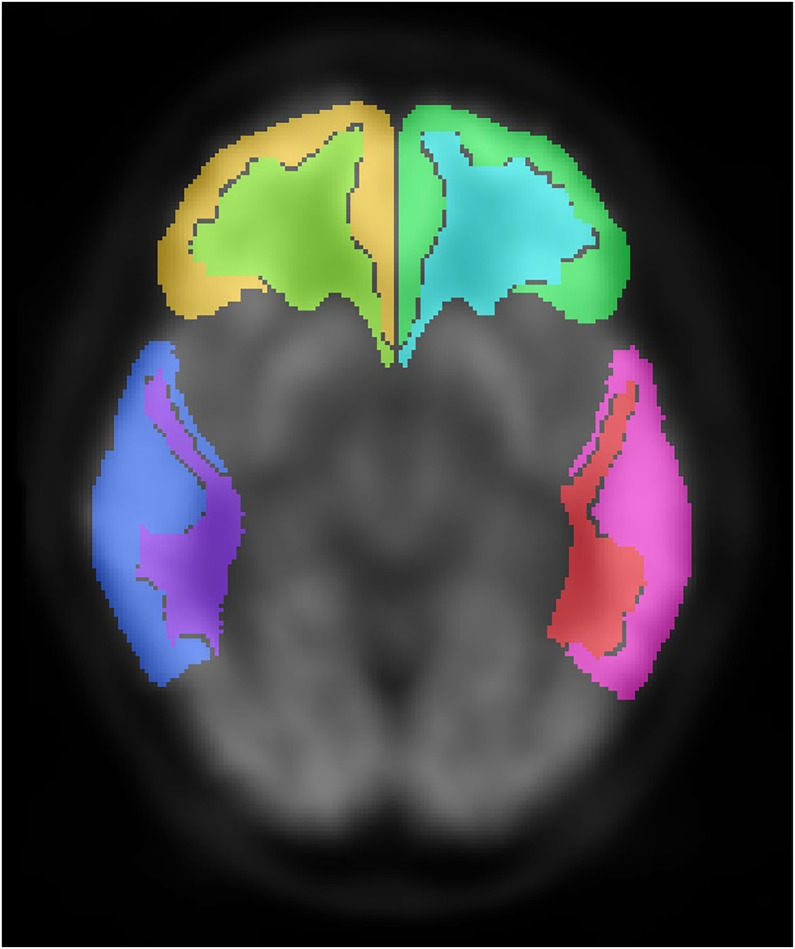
ROI used to assess contrast between white and gray matter. ROI is shown over ^18^F-FDG PET image. Each area is color-coded. ROIs were placed in 4 regions relevant to AD: frontal lobe (yellow, light green, cyan, and dark green), temporal lobe (blue, purple, pink, and orange), parietal lobe, and posterior cingulate gyrus/precuneus. Except for posterior cingulate gyrus and precuneus, ROIs were based on Hammers ROI. Gray matter ROIs in posterior cingulate gyrus and precuneus were created using Automated Anatomic Atlas 3 template, while white matter ROIs were created manually.
